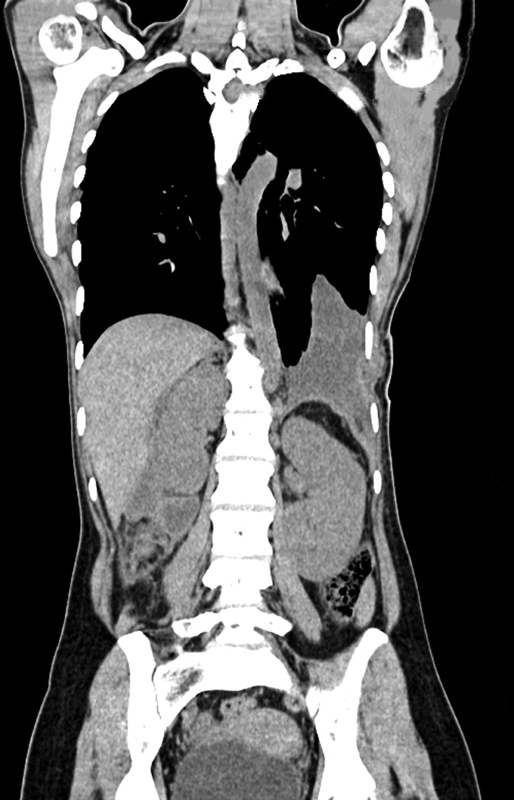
Postoperative contrast-enhanced computed tomography showing neo-diaphragm with minimal pleural effusion and no recurrence at 14 days.
